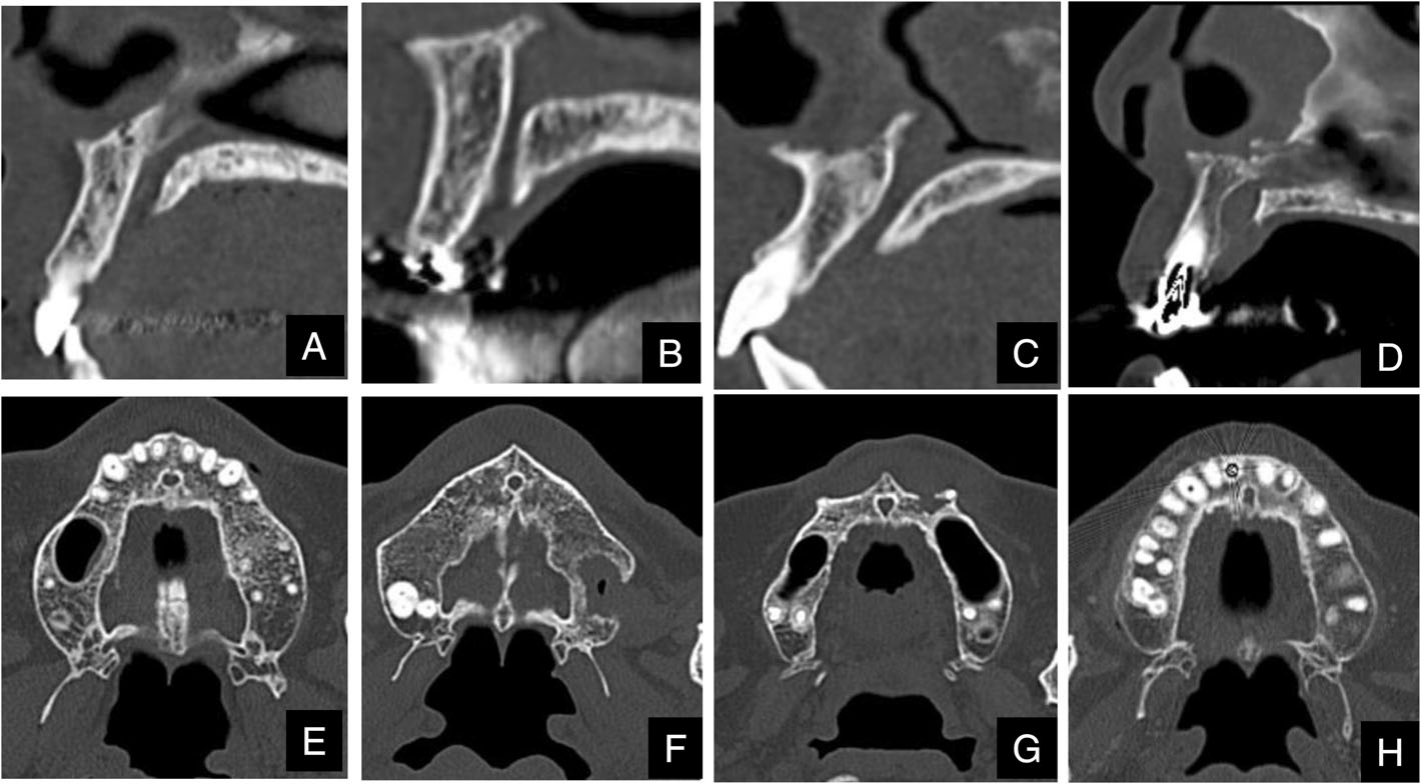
The shapes of normal maxillary incisive canals on CT divided into 4 categories. Based on CT sagittal sections of maxillary incisive canals, they are classified as cylindrical (a), funnel (b), spindle (c), or hourglass (d), and based on CT axial sections, they are round (e), oval (f), heart (g), or others (h)
